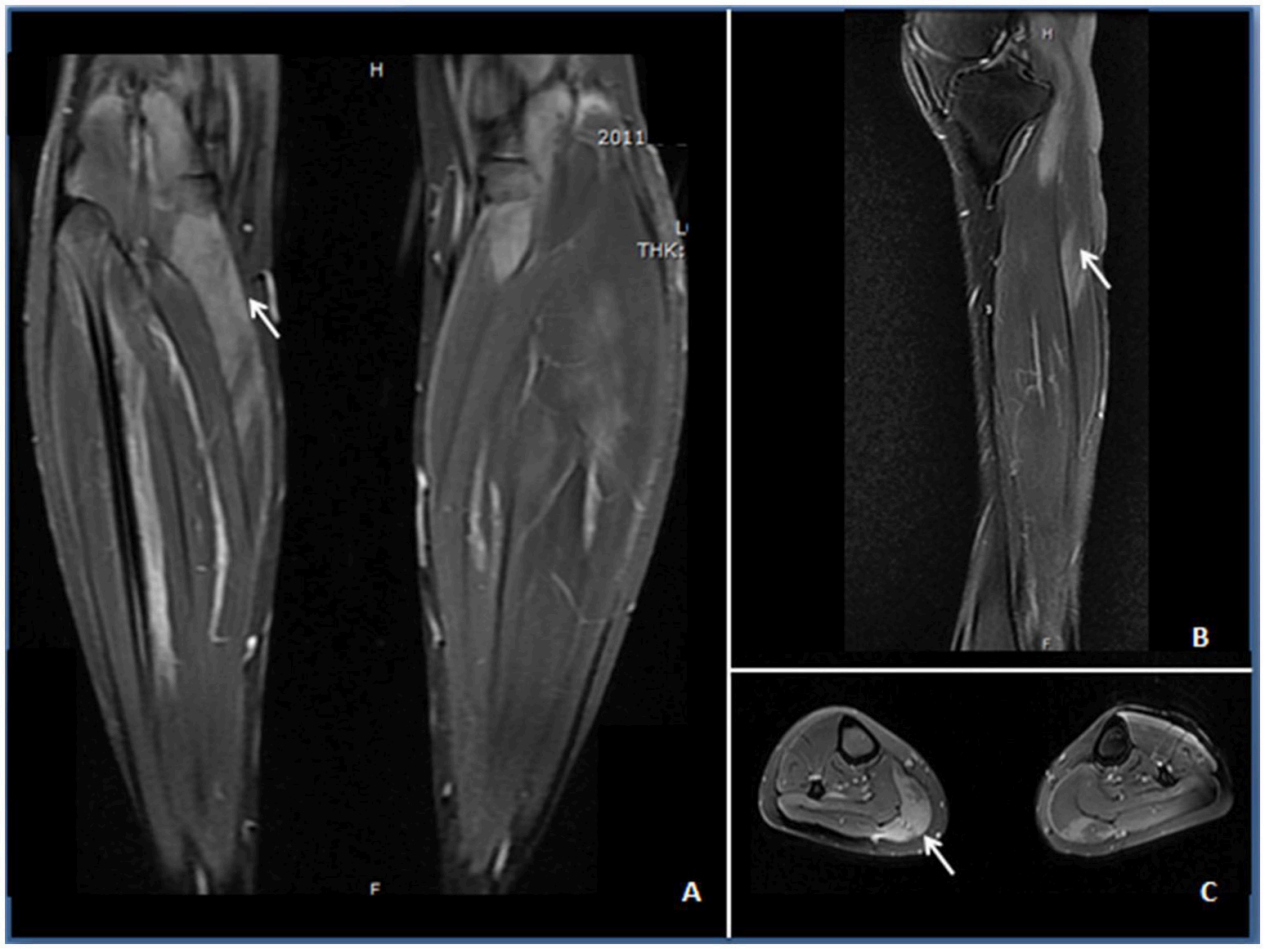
STIR (A) and T2-weighted images (B,C) demonstrating edema (↑) in the anterior and posterior calves of the patient. (A) Increased STIR image signaling in the gastrocnemius with unsymmetrical involvement. (B) Increased intramuscular T2 image signaling within the anterior tibial muscle at the sagittal section. (C) Patchy T2-weighted hyperintense area in the gastrocnemius, soleus, and anterior tibial muscles.
